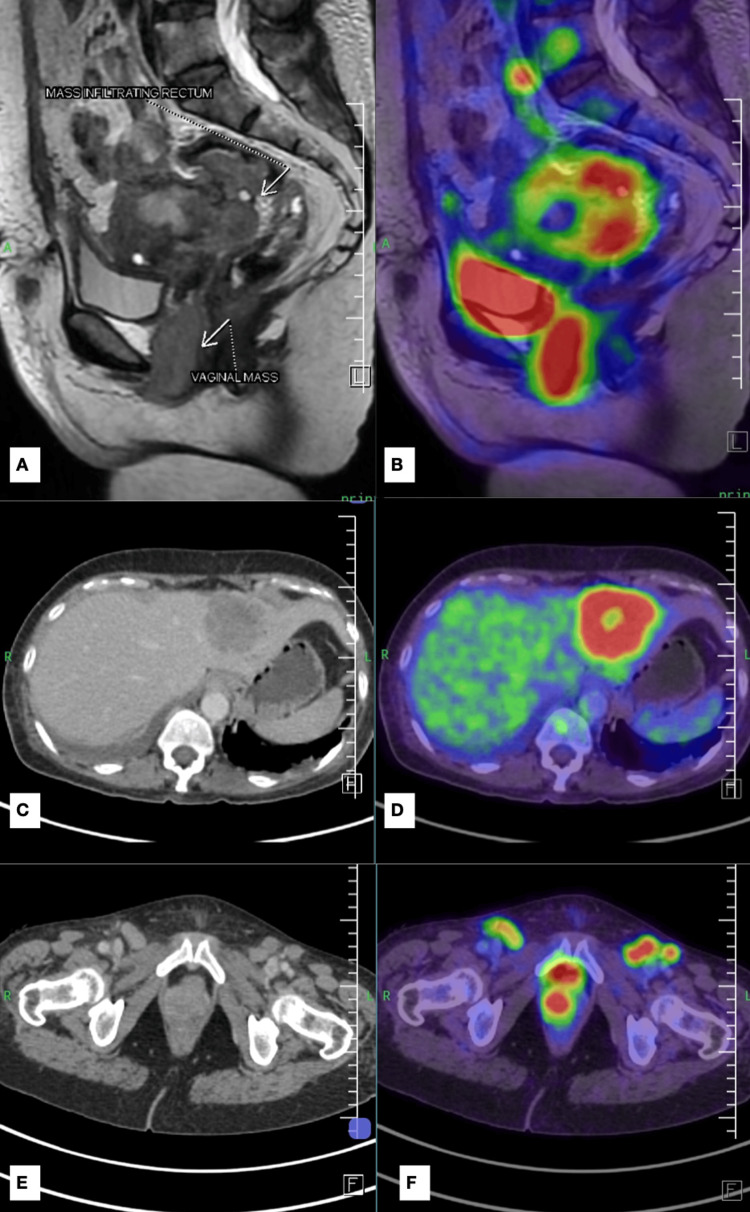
Postoperative positron emission tomography - computed tomography A) Sagittal T2-weighted (T2W) images show a relatively well-defined hyperintense mass lesion in the vagina. There is an ill-defined heterogeneous infiltrative mass lesion involving the upper 1/3rd of the rectum and distal sigmoid colon; B) These above-mentioned lesions show FDG avidity on the PET CT; C) Axial CT image showing a relatively well-defined heterogeneously enhancing lesion in the left lobe of the liver suggestive of metastasis; D) PET-CT image showing the same relatively well-defined heterogeneously enhancing lesion in the left lobe of the liver showing fluorodeoxyglucose (FDG) avidity suggestive of metastasis; E) Axial CT images showing mass lesion in the vagina with multiple enlarged bilateral inguinal lymph nodes; F) Axial PET CT images show FDG-avid mass lesion in the vagina and multiple FDG avid bilateral inguinal lymph nodes
